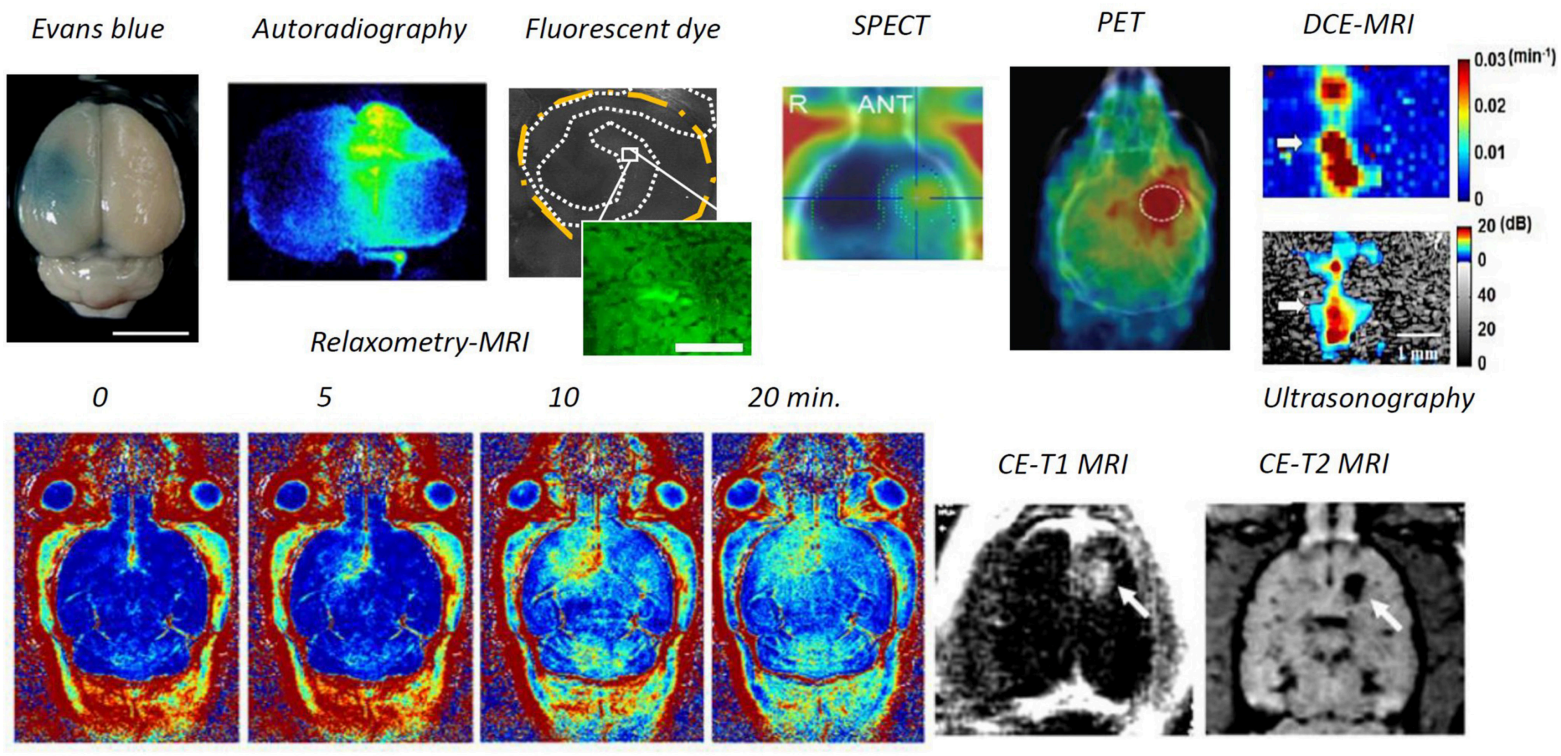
Modalities to identify BBB opening. Through *ex-vivo* examination, Evans blue dye can directly depict the BBB-opened region from gross section, or fluorescent dextran or the radioactivity readout through autoradiography from the brain gross section can be used to identify the BBB-opened region. Previous attempts have included *in-vivo* examination, ultrasonography via microbubble dynamic characterization, SPECT/ PET via radiotracer, contrast-enhanced MRI either via Gd-DTPA or MNPs), and dynamic contrast-enhanced MRI via Gd-DTPA (Lin et al., 2009; Liu et al., 2009, 2010a, 2016; Chai et al., 2014; Fan et al., 2014; Xia et al., 2016; Wu et al., 2017).
